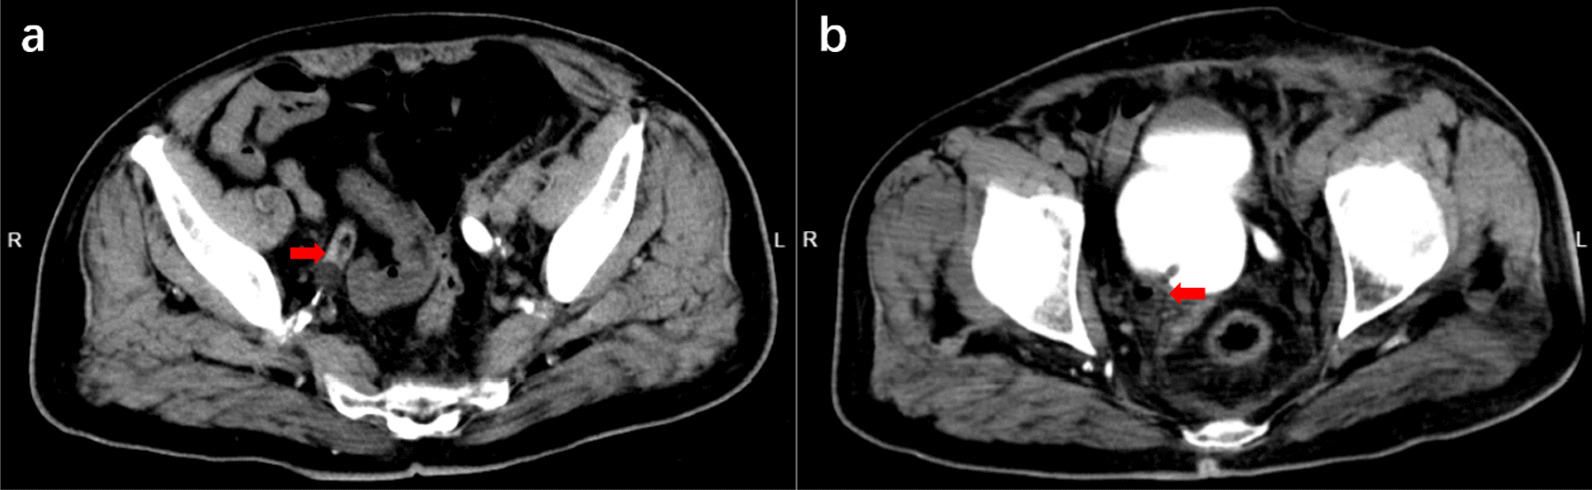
Images from computed tomography. a The tip of the Foley catheter is located in the right lower ureter. b The saline-filled balloon in the ureter cavity
